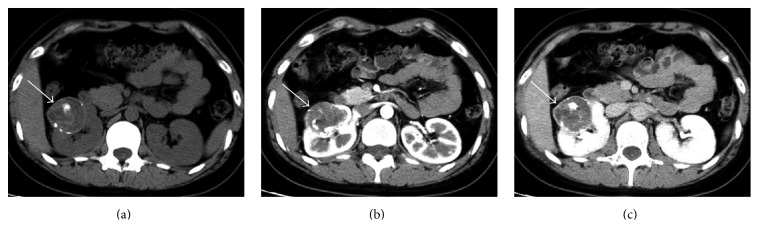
Renal cell carcinoma associated with Xp11.2 translocation/TFE3 gene fusions in a 22-year-old woman with gross hematuria. (a) Abdominal plain CT scan shows a round well-defined mass of 5.0 cm in size with mixed intensity (around 50 HU) in the right kidney. Note the punctate and circular calcification within and around the lesion. (b) The tumor, which involves both the cortex and medulla, shows heterogeneously moderate enhancement (to around 84 HU) during corticomedullary phase. (c) The tumor continues to be enhanced heterogeneously from corticomedullary phase to nephrographic phase (to around 90 HU). The unenhanced areas within the tumor indicate necrosis.
